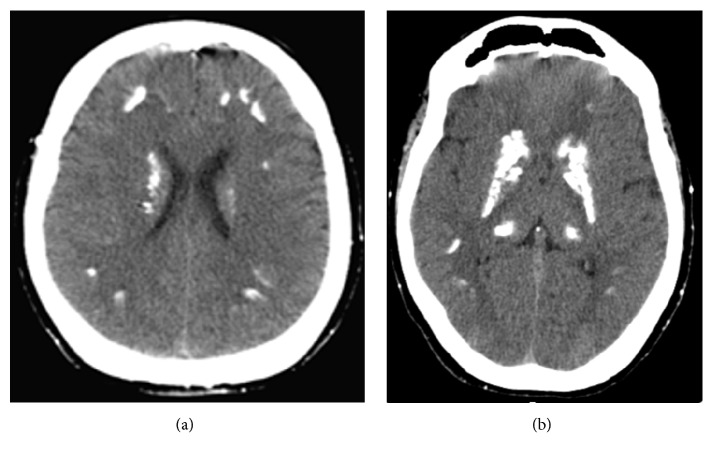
Axial cuts of CT scan, revealing diffuse subcortical frontoparietal (a) and striatum capsularis and thalamus calcifications (b).
